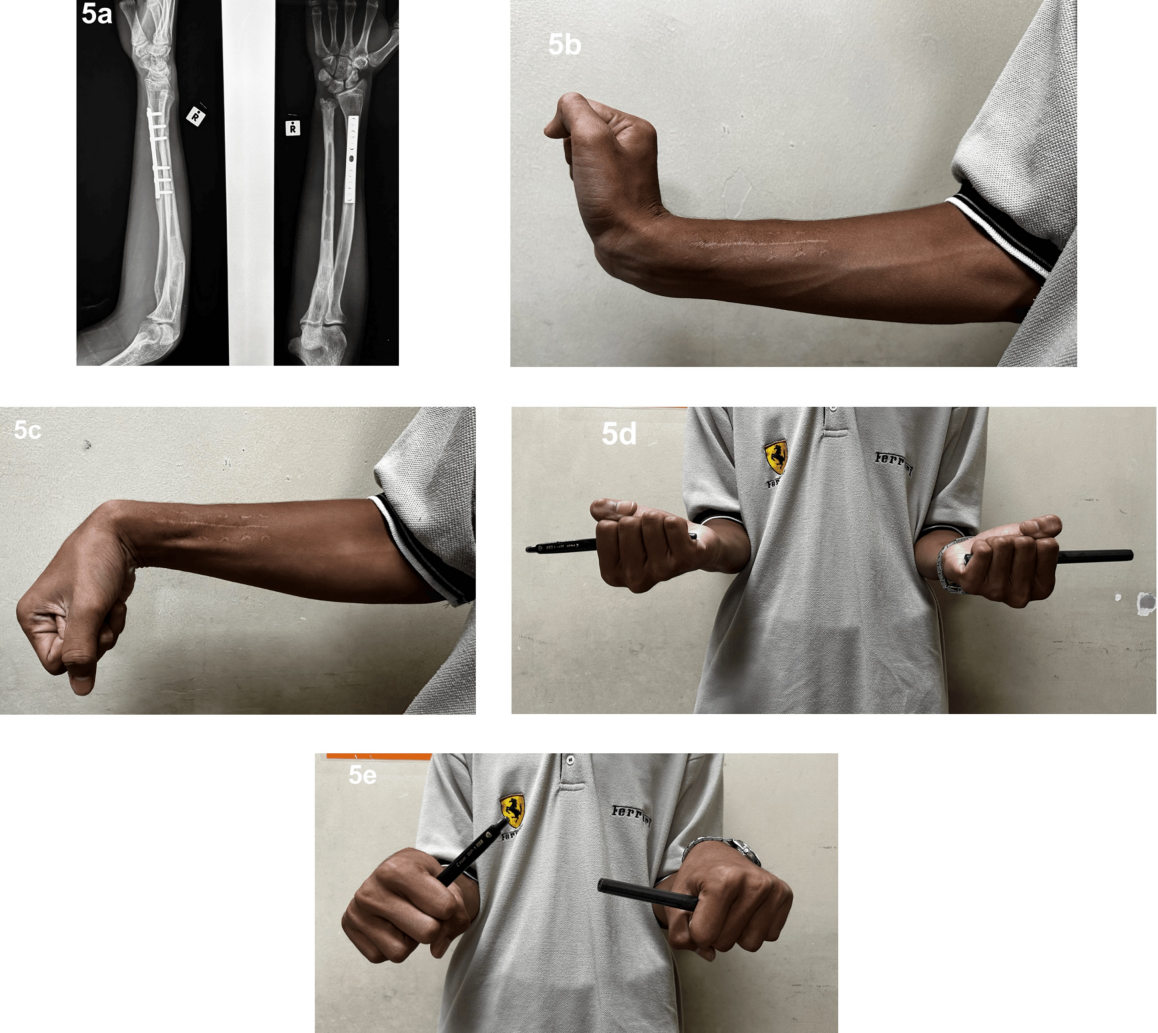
Postoperative radiograph in anteroposterior and lateral views demonstrating solid bony union with satisfactory anatomical alignment (5a); clinical image showing wrist extension up to 80 degrees (5b); wrist flexion up to 70 degrees (5c); full supination up to 90 degrees (5d); and pronation up to 45 degrees (5e).
